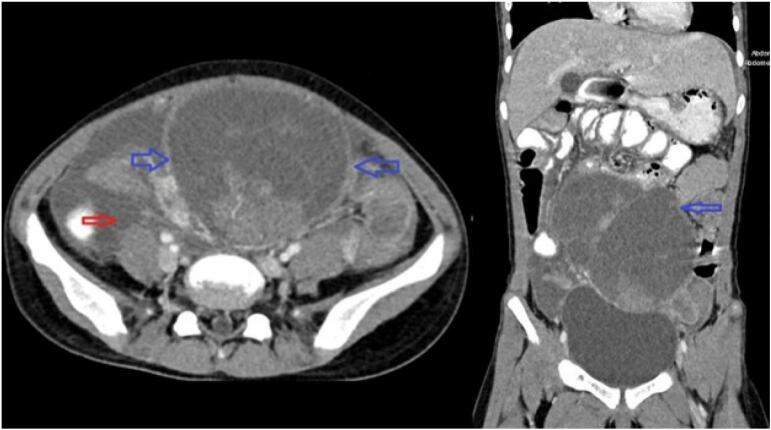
Axial and coronal views of a contrast-enhanced CT scan (IV and oral contrast) demonstrating a well-defined solid-cystic mass in the midline of the lower abdominal cavity, situated superior to the urinary bladder (blue arrows). The axial view also reveals the presence of ascites (red arrow). (For interpretation of the references to colour in this figure legend, the reader is referred to the web version of this article.)
